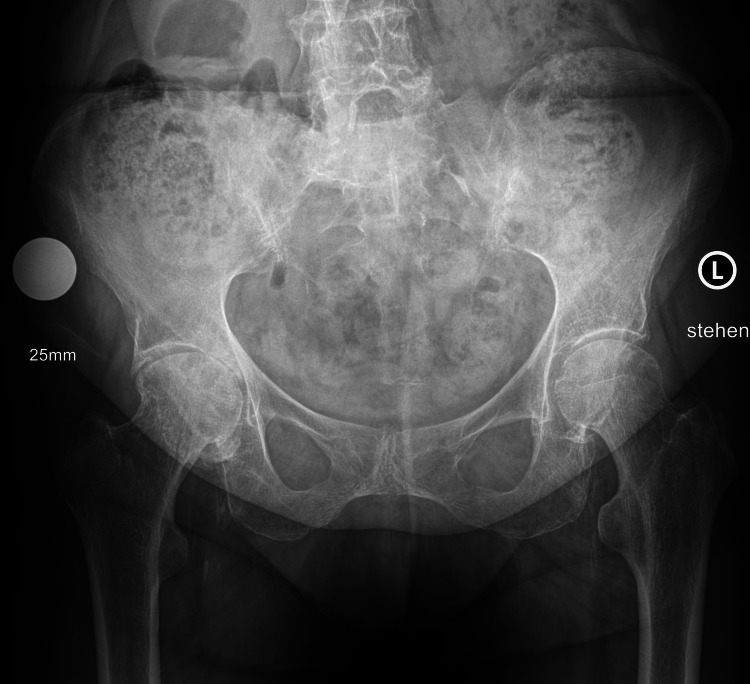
Preoperative radiographs before implantation
